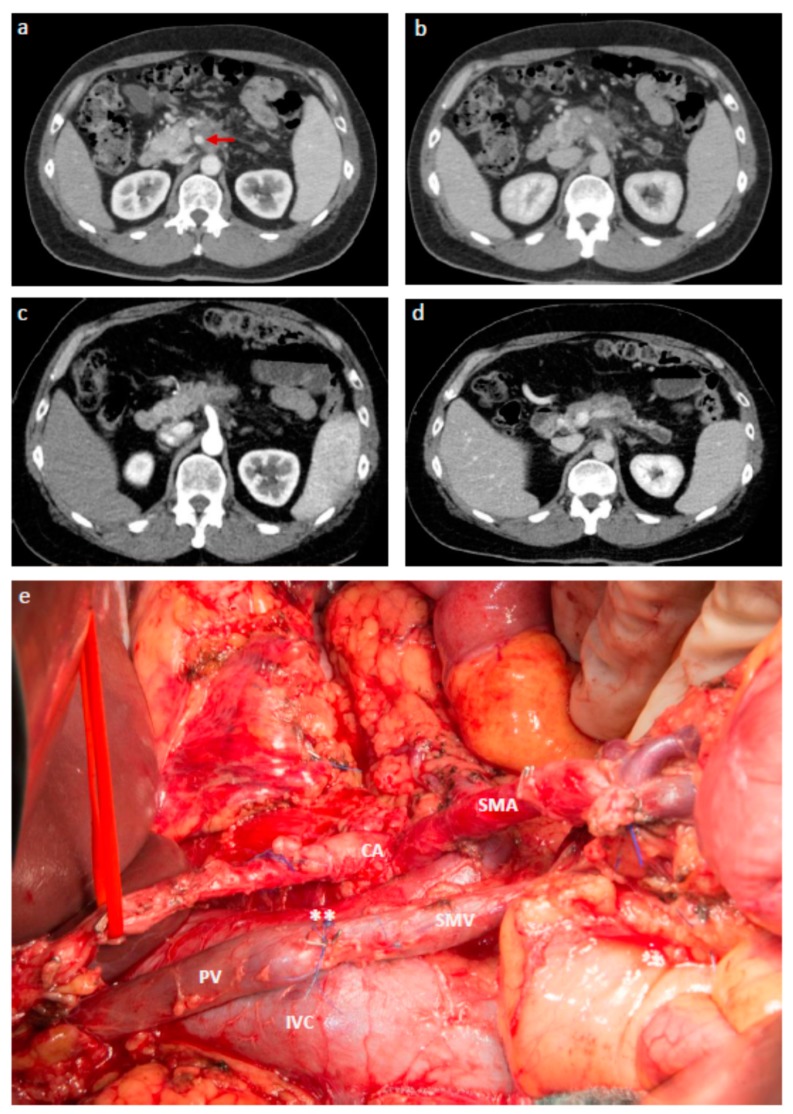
LAPC with partial response after NAT receiving extended tumor resection with perivascular dissection: a–d: Transverse multidetector contrast enhanced CT images taken before initiation of NAT ((a) arterial phase; (b), venous phase) and after completion of NAT with three cycles of FOLFIRINOX and 11 cycles of FOLFIRI (c, arterial phase; d, venous phase). Unresectable PDAC of the pancreatic body with encasement of the SMA (red arrow in a) and cavernous transformation of the portal vein (b). Partial response on CT (c and d). (e): Operative site after extended tumor resection with artery first, total pancreatectomy, splenectomy, adrenalectomy, subtotal gastrectomy, subtotal colectomy, TRIANGLE-operation with PV/SMV resection () and perivascular level-III dissection around the SMA and celiac axis (CA). Final pathology showed ypT2, ypN1 (1/49), L0, V0, R0. The patient had a peritoneal recurrence at 24 months after resection.
